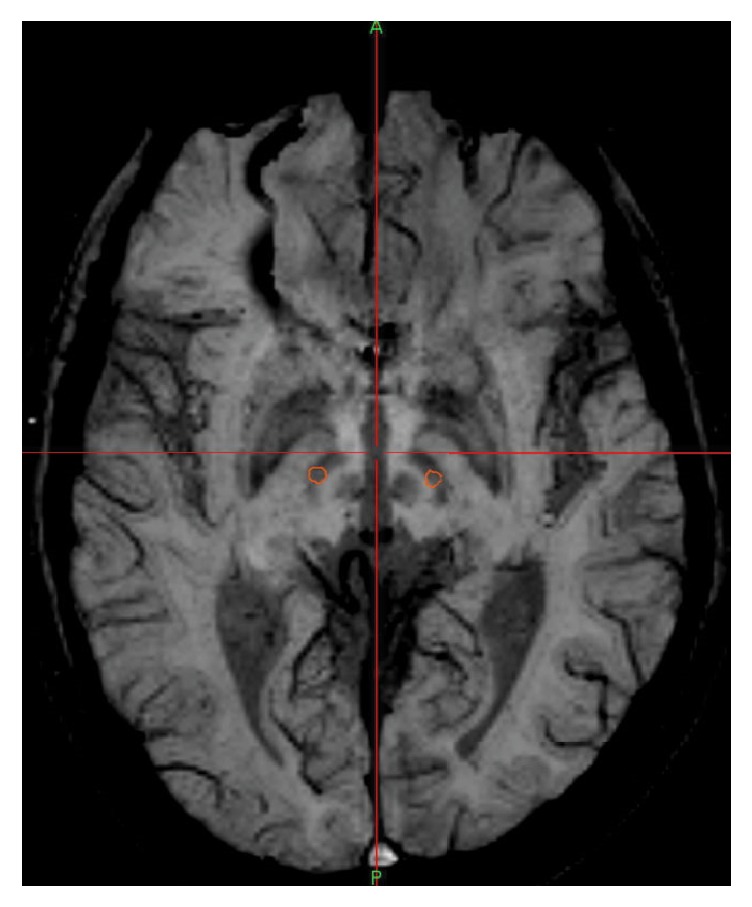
Merging SWI sequence with postoperation CT to determine the electrode position.
